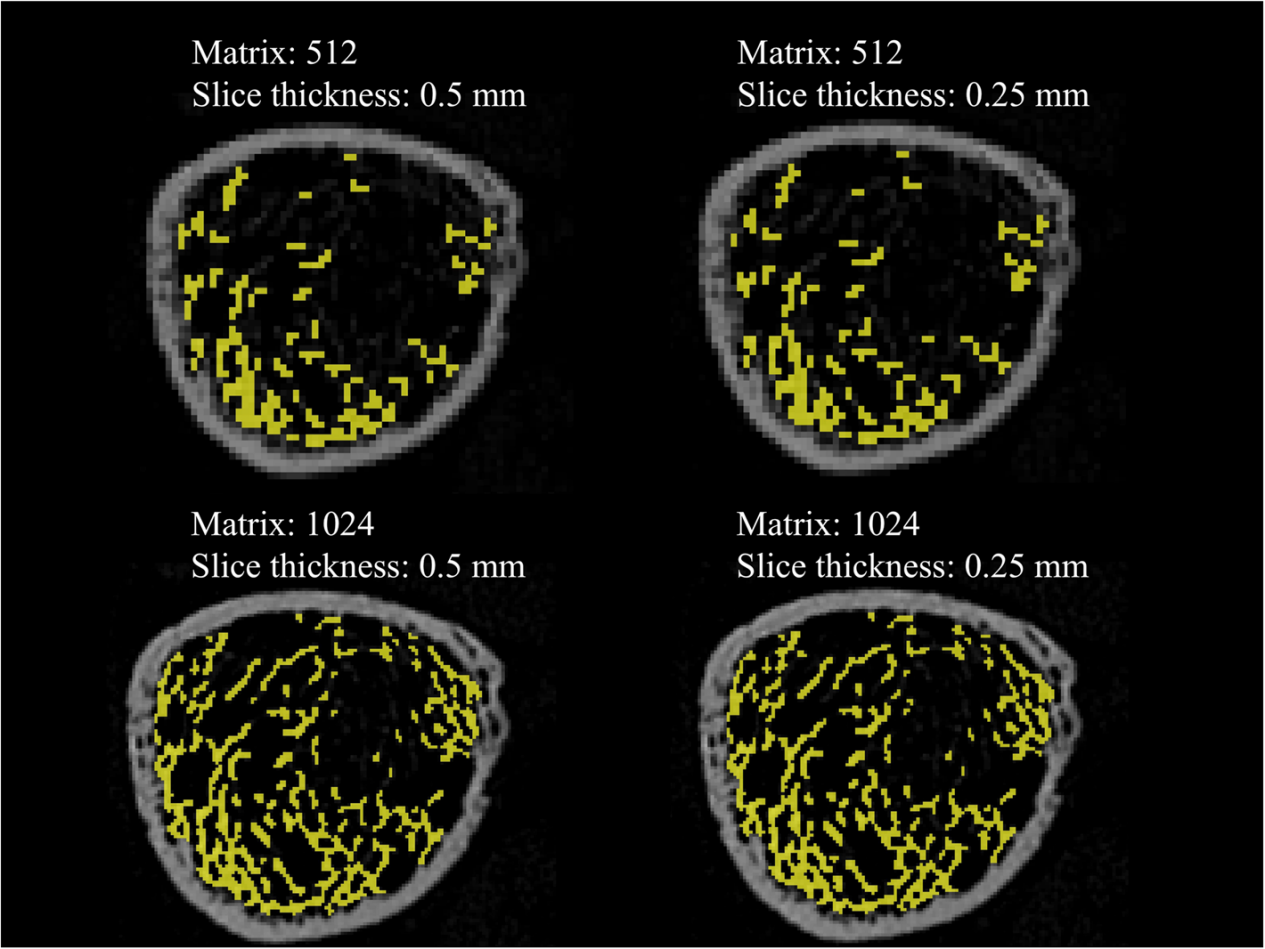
Extracted images representative of the trabecular bone in the human distal tibia on ultra-high-resolution computed tomography (UHRCT). The matrix numbers were 512 × 512 and 1024 × 1024 and the slice thicknesses were 0.5 mm and 0.25 mm
